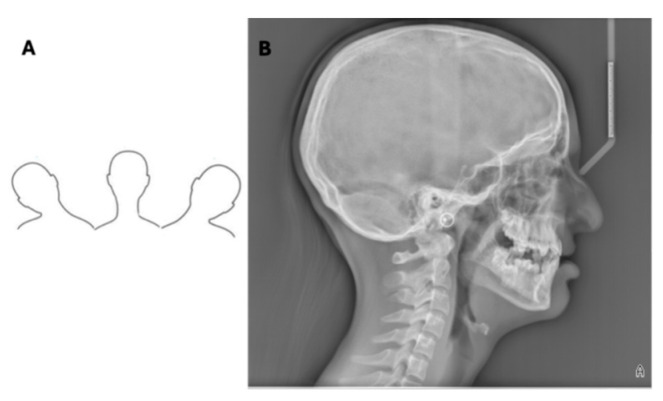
Tilting. (A) Picture. (B) LCR performed with hybrid device. Head tilt results in asymmetric projection of craniofacial structures of the right and left sides. These structures are not overlapping on the radiograph but misaligned in the cranio-caudal direction. Such misalignment is evident at the molar teeth and mandibular bone profiles. In addition, note the open positioning of the lips. Acquisition parameters: 90 kVp, 15 mA, 0.8 s.
